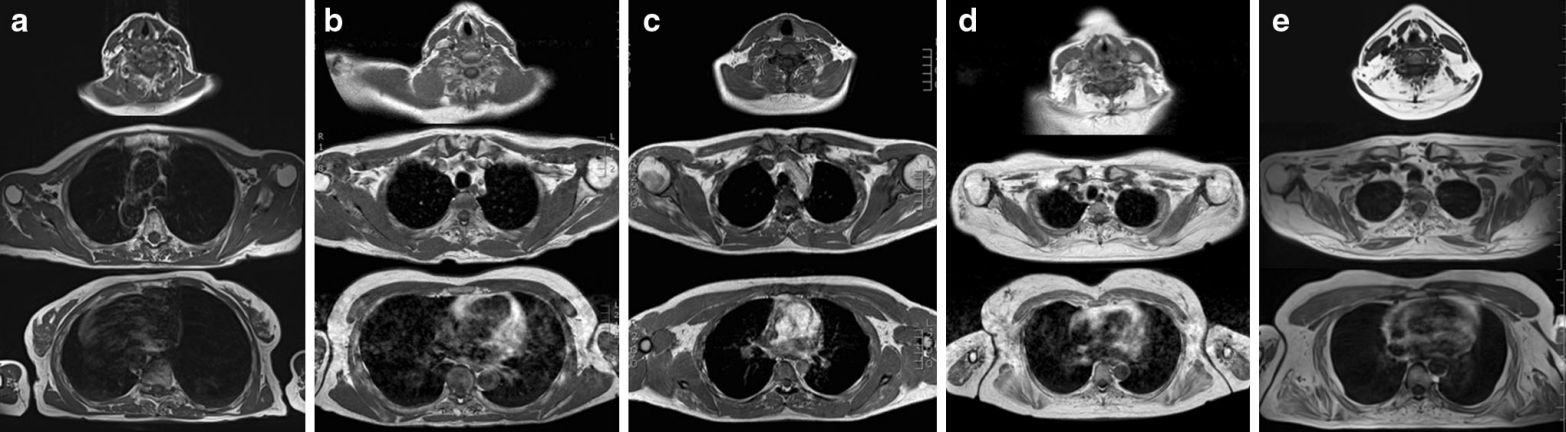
Scapular girdle involvement in NLSD-M. T1-weighted images of lower limbs in NLSD-M patients of different severities: a P10, 74 years; b P1, 62 years; c P9, 25 years; d P6, 52 years; e P4, 45 years. Infraspinatus, trapezius, deltoid and thoracic paraspinous were the most affected muscles and subscapularis, pectoralis minor and major, and sternocleidomastoid were the most spared. Pectoralis major and sternocleidomastoid sometimes had a hypertrophic appearance (e). Note that least affected patients (P10, a; P1, b) showed no T1-hyperintense muscle in scapular girdle. Interestingly infraspinatus showed STIR positive signal in P1 (b) (see Fig. 4)
